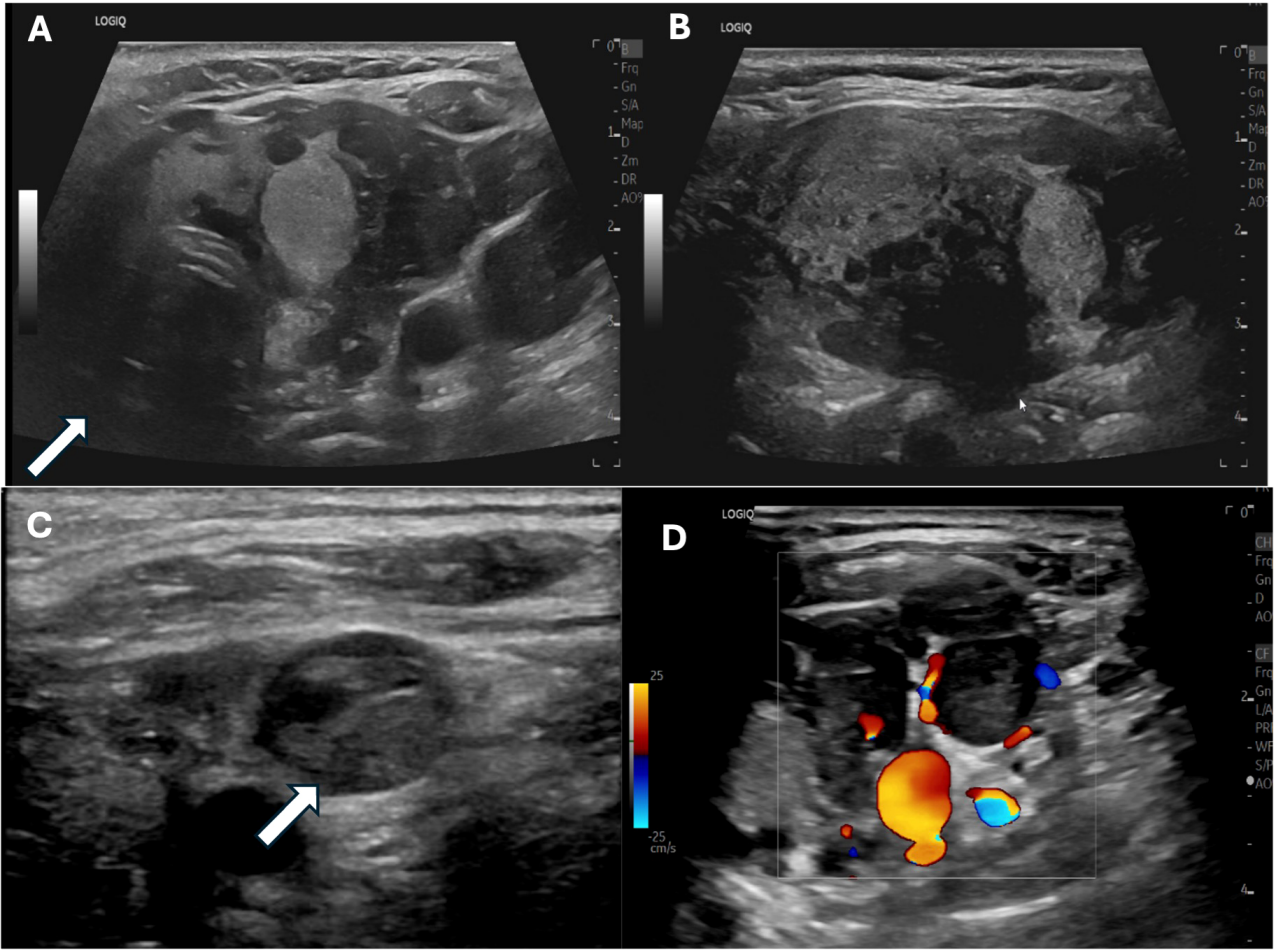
Preoperative sonogram of the left central neck (A), transverse plane; (B), longitudinal plane) demonstrating the multinodular left thyroid lobe with an arrow indicating the heterogeneous dominant left thyroid nodule replacing most of the left lobe, which returned benign cytology on fine needle aspiration. Preoperative neck ultrasound image (C) demonstrates the left internal jugular vein (white arrow) with intraluminal thrombus devoid of internal flow on doppler imaging (D).
